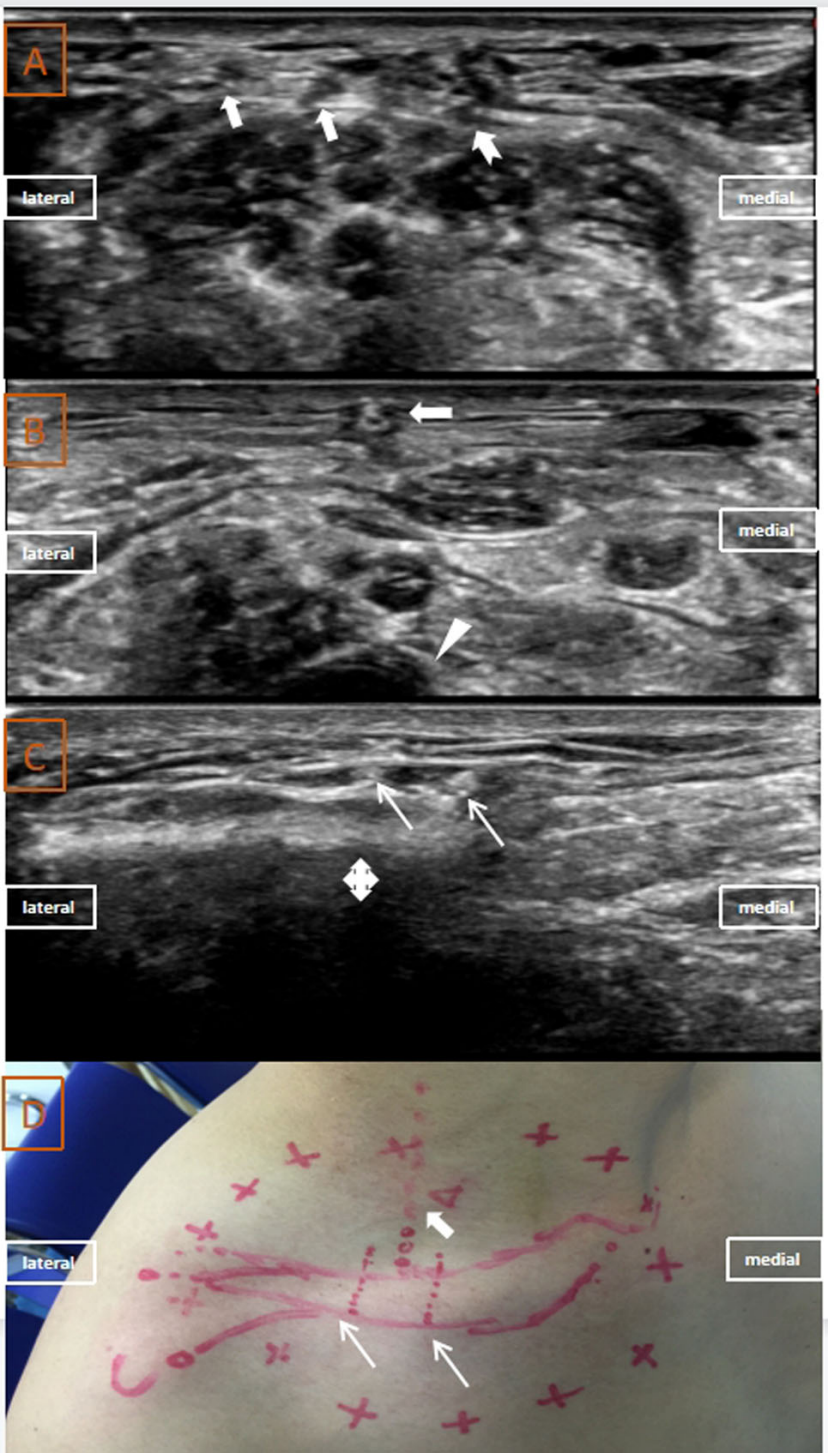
Ultrasound images, projection on the body surface and sensory block area of the supraclavicular nerve in Case 1: (A) ultrasound images of phrenic nerve (indicated with swallowtail arrow) and superficial cervical plexus (indicated with a thick white arrow); (B) ultrasound image of the supraclavicular nerve (indicated with a thick white arrow) anterior to the supraclavicular artery (indicated with a white arrowhead); (C) ultrasound images of branches of the supraclavicular nerve (indicated with thin white arrows) anterior to the clavicle (indicated with a cross arrow); (D) projection of the supraclavicular nerve on the body surface (indicated with a white arrow) and the sensory block area (red circle).
